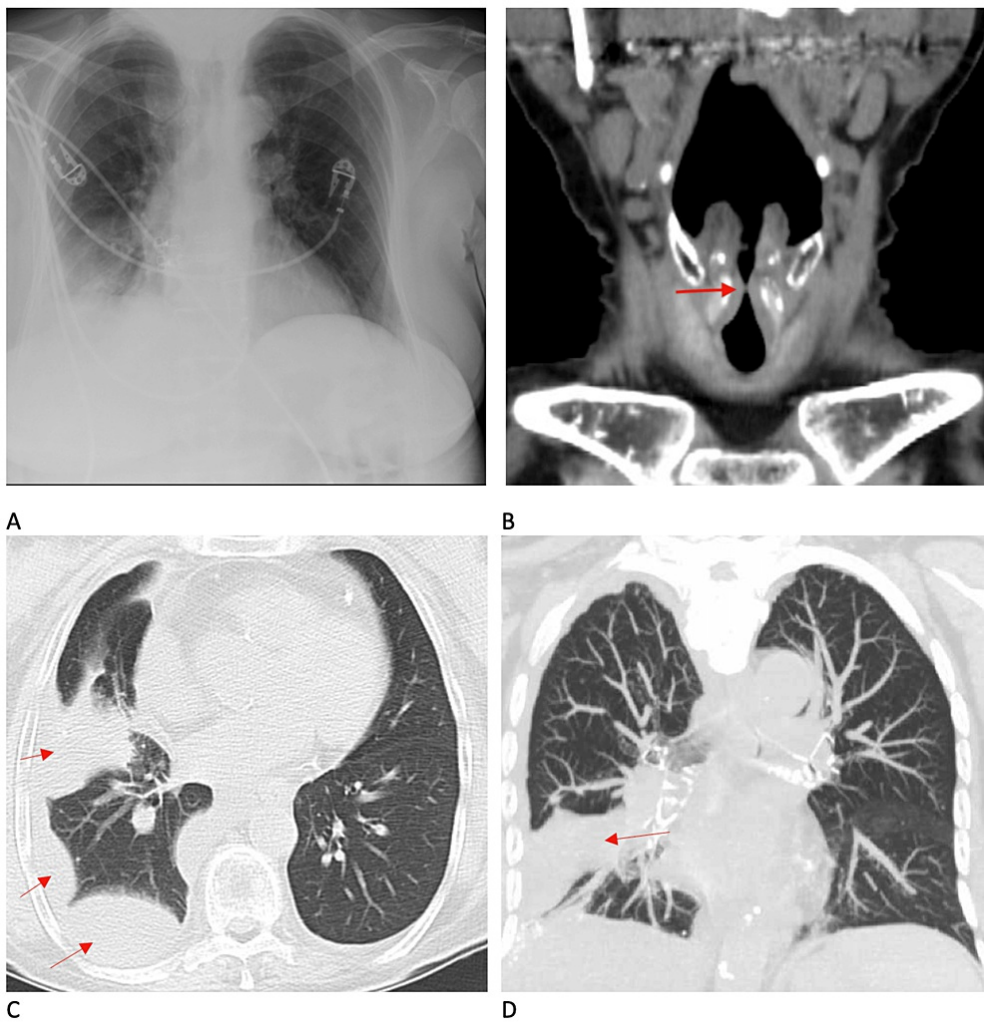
Imaging of the patient's chest and neck A. Chest X-ray demonstrating small right pleural effusion and adjacent atelectasis B. CT scan of the neck demonstrating subglottic stenosis below the level of the vocal cords (dark red arrow) C. CT scan of the chest, coronal view, demonstrating complex fluid collections in the right lung (red arrows) D. CT scan of the chest, axial view, demonstrating complex fluid collections in the right lung (red arrow)
